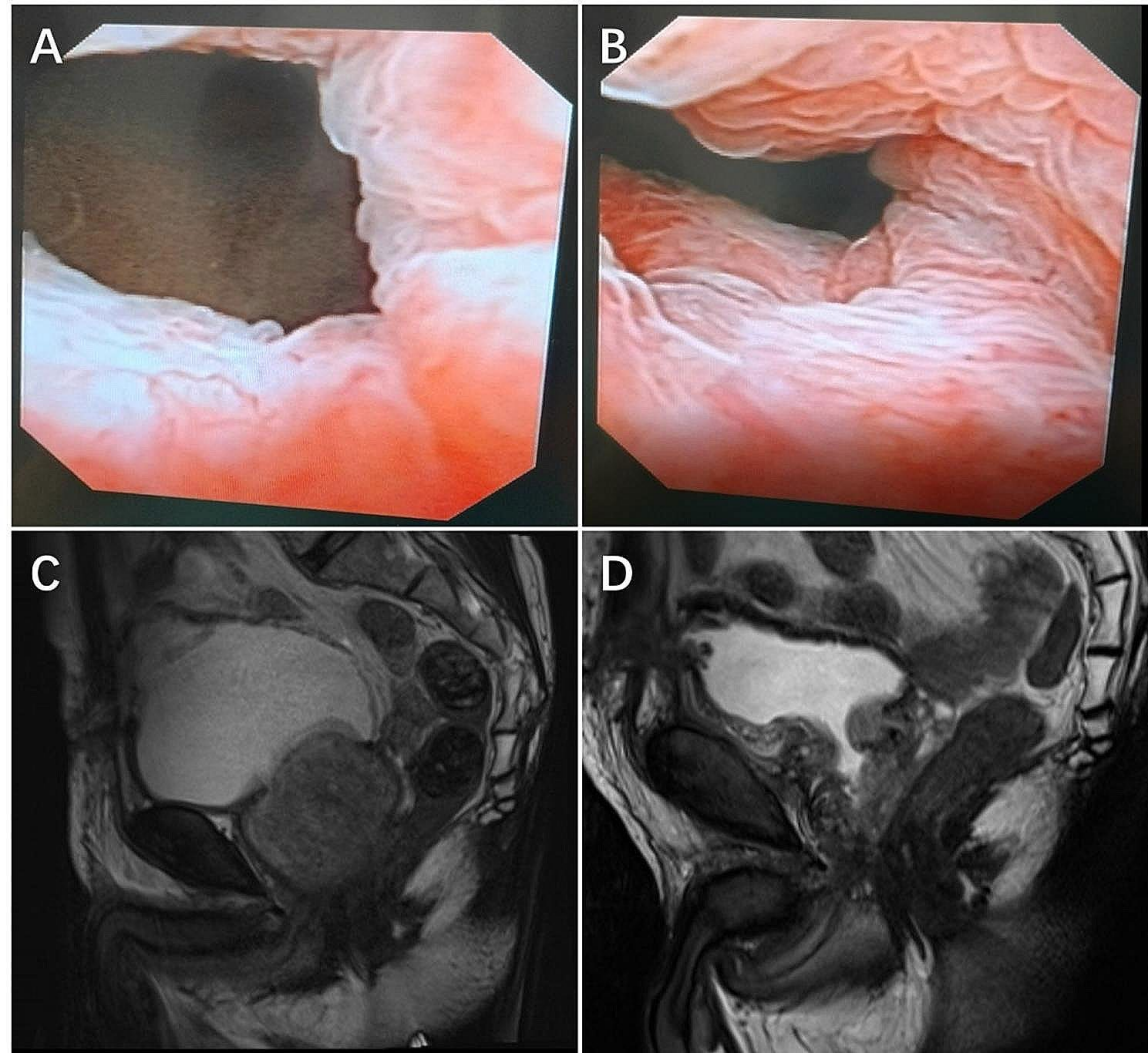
A and B, Postoperative flexible cystoscopy revealed that bladder neck and urethra were unobstructed, and the mucosa at bladder neck and prostatic urethra was intact; C, Preoperative mp-MRI showed a large and obstructive prostatic adenoma; D, postoperative mp-MRI revealed that the grands were completely removed and urethras were unobstructed and intact
